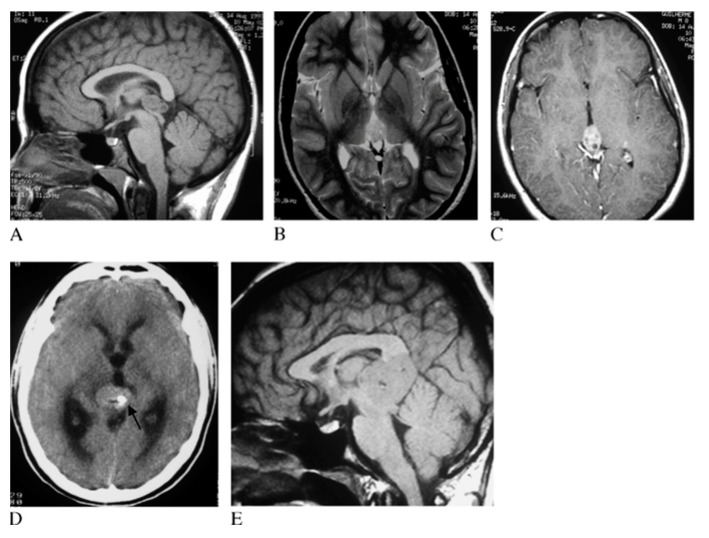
Pineal germinoma magnetic resonance imaging (MRI). Pineal tumor hypointense on T1 weighted image (T1WI) (A), hyperintense on T2WI (B) and with homogeneous contrast enhancement (C). The arrow identifies hyperdense mass with calcification at computed tomography (D). In another patient, a larger germinoma, isointense on T1WI (E). Reprinted with permission from Reis et al. (2006) [41]. John Wiley and Sons—2021 (License Number 5034711197310).
